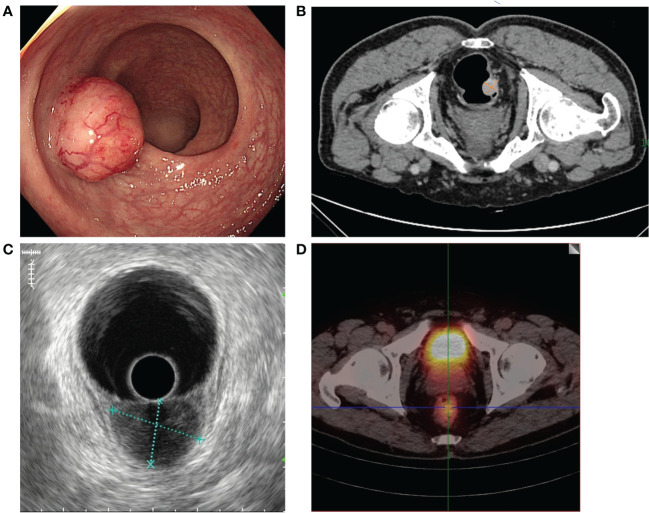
Preoperative endoscopic and imaging examination results. (A) Colonoscopy revealed the tumor was 2.4 cm × 2.0 cm in diameter in the lower rectum with a wide stratum. (B) CT revealed no LLNs enlargement in the mesorectum and on both sides of the lateral pelvic space. (C) The EUS reveals the lesion invades the submucosal layer and no LLNM. (D)
